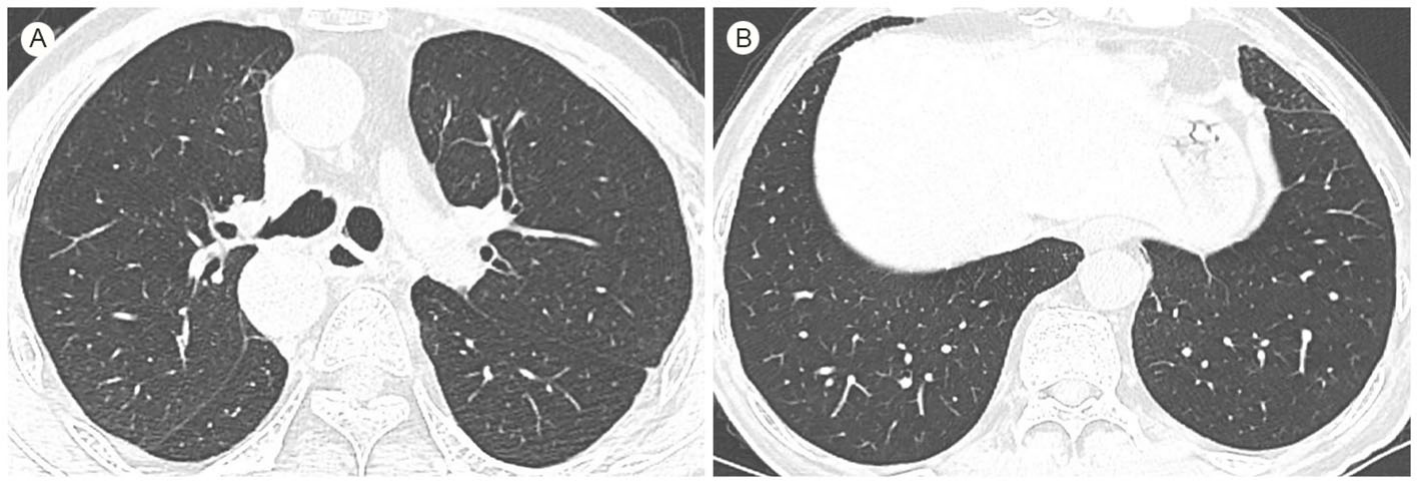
Postoperative HRCT at 3 month: (A, B) good translucency in both lungs.
